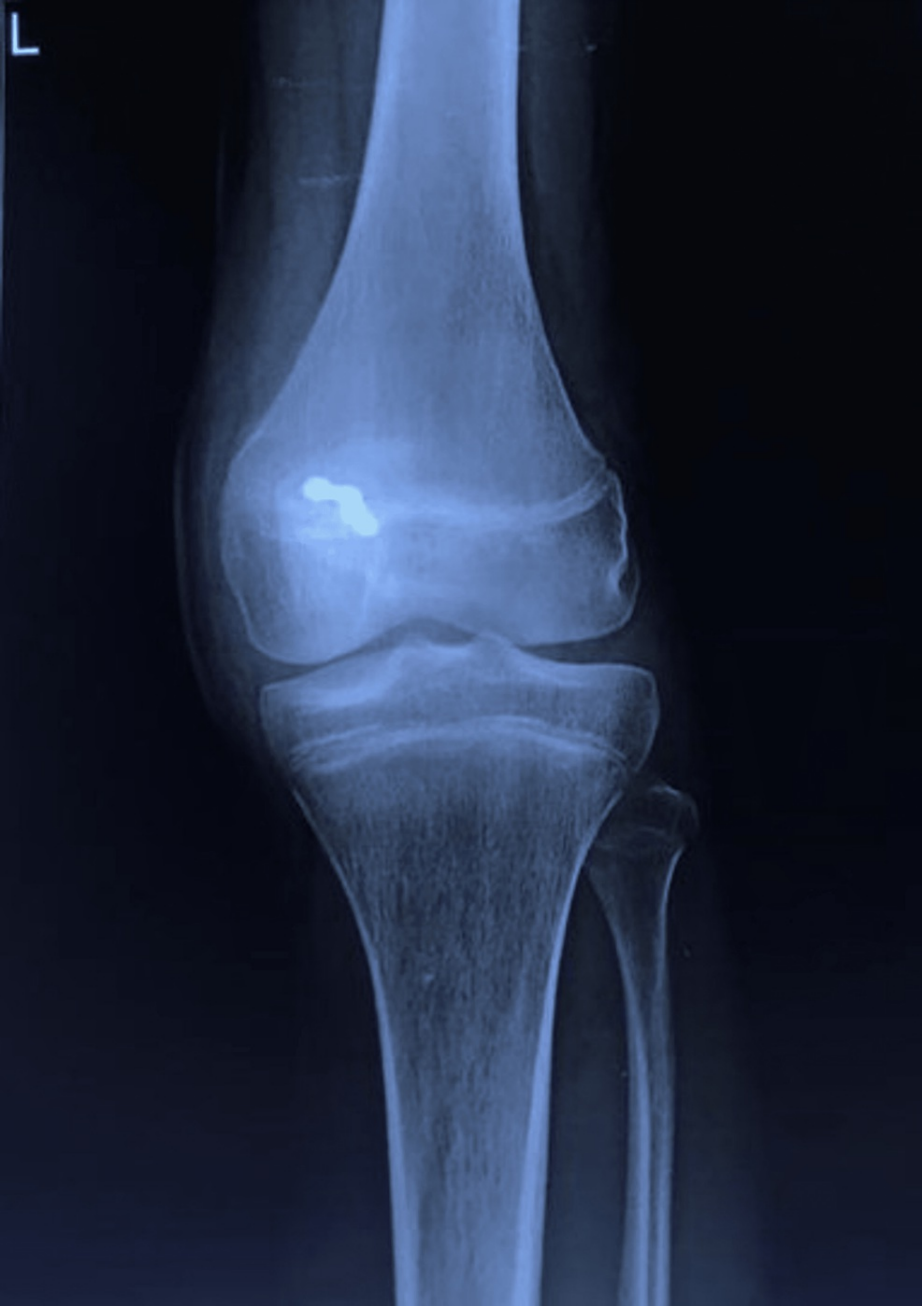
Postoperative anteroposterior roentgenogram
